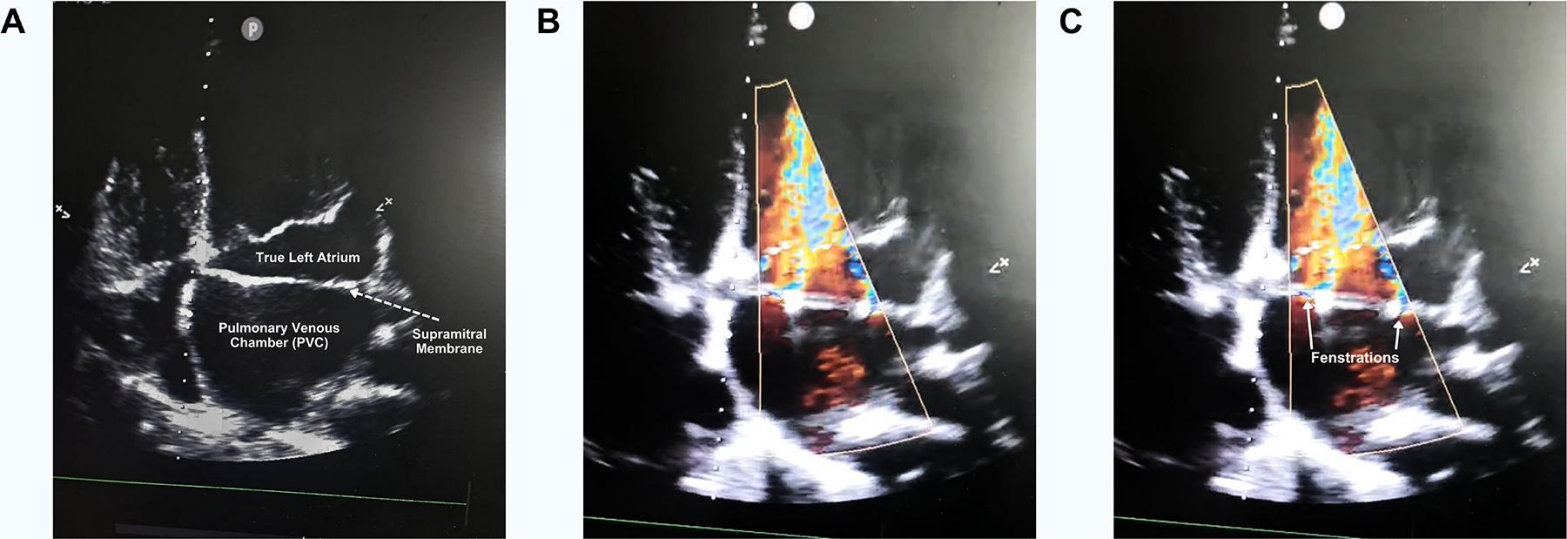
Apical four-chamber transthoracic echocardiographic images demonstrating Cor triatriatum sinister. (A) 2D apical four-chamber view showing a supramitral membrane that divides the left atrium into a posterior–superior pulmonary venous chamber (PVC) and an anterior–inferior true left atrium (LA); the true LA lies between the membrane and the mitral valve and communicates directly with the left ventricle (LV). (B) Color Doppler apical view demonstrating flow jets traversing multiple fenestrations in the membrane, indicating residual communication between the PVC and true LA. (C) Same color Doppler image as panel B, annotated with white arrowheads that mark the two principal fenestrations.
